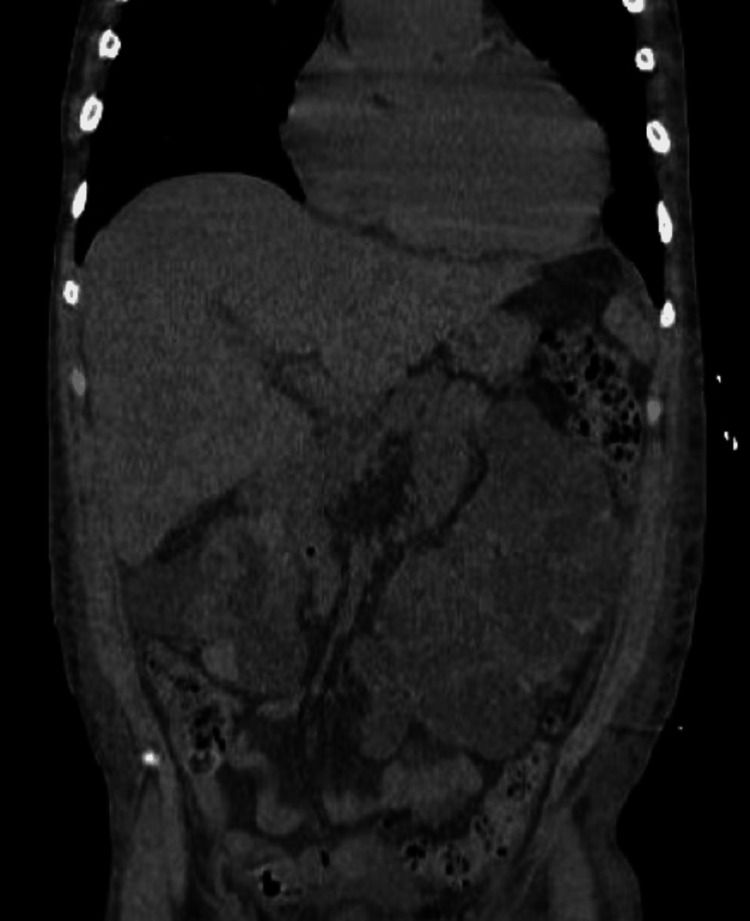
Contrast-enhanced CT scan of the abdomen and pelvis performed to rule out post-surgical and cystic complications revealing multiple renal and hepatic cysts with no evidence of infection, bleeding, or obstruction CT: computed tomography
